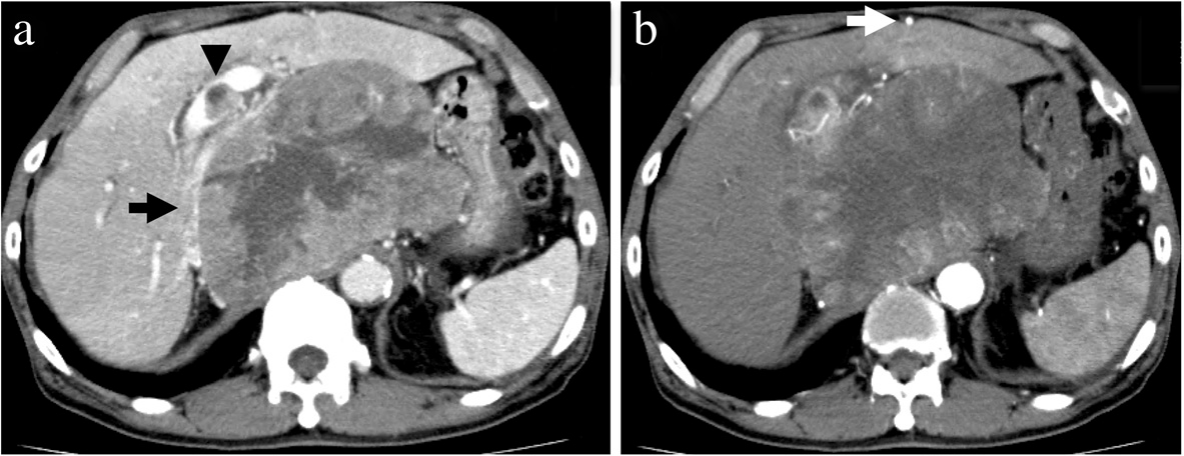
Enhanced abdominal computed tomography results. (a) Enhanced abdominal computed tomography (CT) showed a large solitary tumor measuring 15 cm in diameter occupying the whole caudate lobe, subsequently pushing up the inferior vena cava (IVC) ventrally (arrow). In addition, a tumor thrombus (2 cm in diameter) was found at the bifurcation of the portal vein (arrowhead). (b) Enhanced CT showed that the right gastroepiploic artery graft passed through the antehepatic route (arrow).
